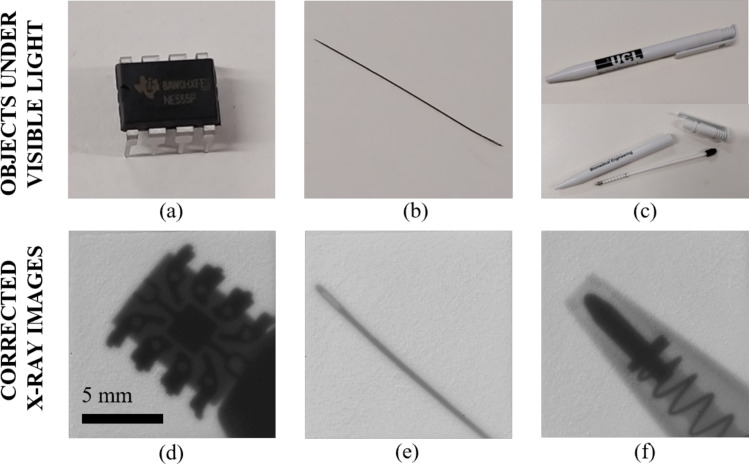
Imaging of an electronic chip, a needle, and a pen containing a spring mechanism using the imaging skin system (left to right). a–c show the objects as they appear under visible light and d–f are the X-ray images after noise corrections. The imaging skin system is able to resolve internal structures that cannot be seen under visible light
